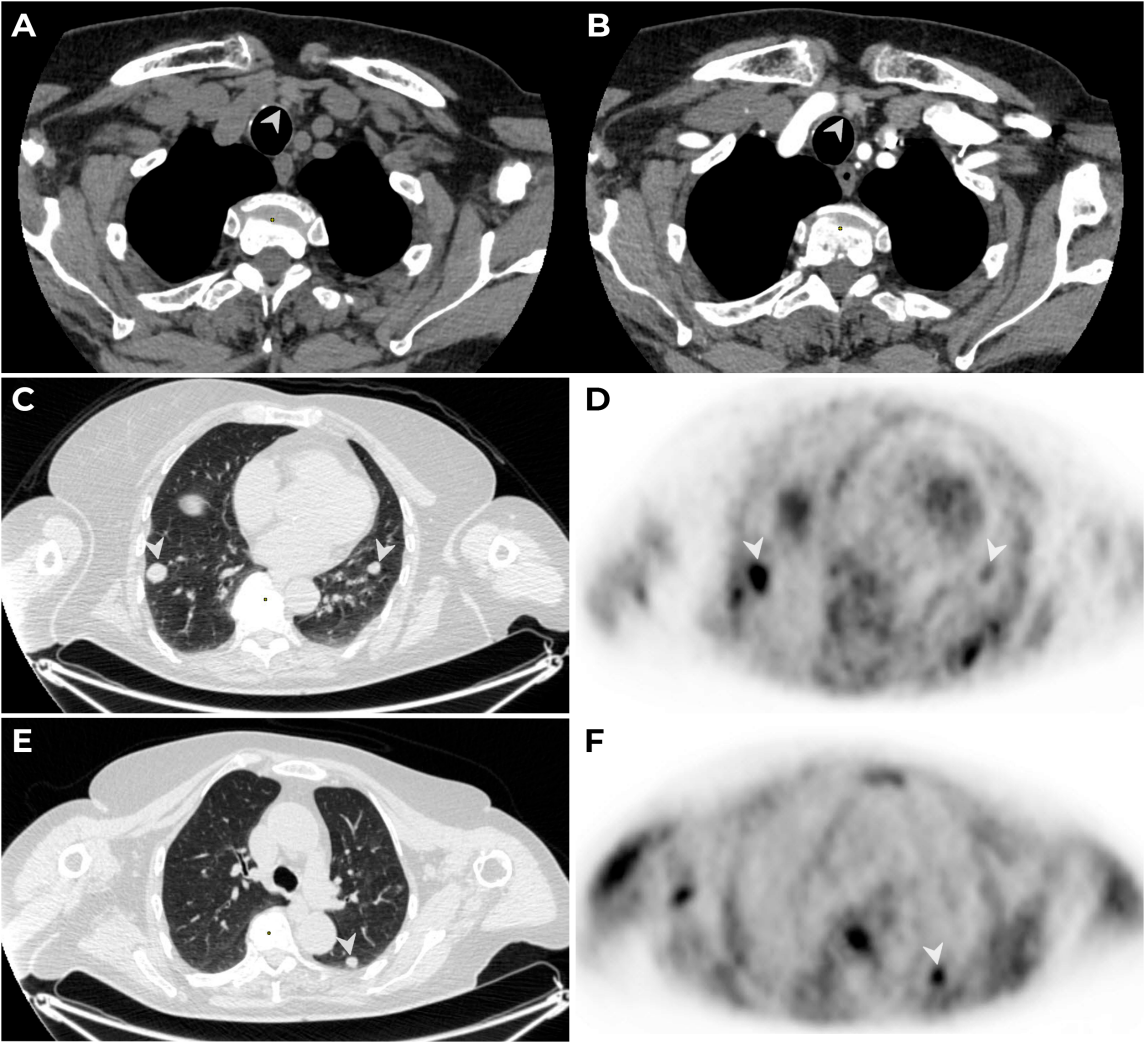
MSCT: native (A) and arterial (B) phases of contrast enhancement. A lesion with an oval shape, smooth clear contours, 6 mm × 5 mm × 5 mm in diameter (native density: 83 HU, 104 HU in the arterial phase and 93 HU in the venous phase) is detected paratracheal to the left (→) along the lower contour of the left lobe of the thyroid gland. PET/CT with ^11^C-choline. The CT thorax (C, D) visualizes secondary lesions (mts) (→) in S9 of the right and left lungs (C, D) and in S6 of the left lung (E, F), with hyperfixation (→) of the radiopharmaceutical on PET.
